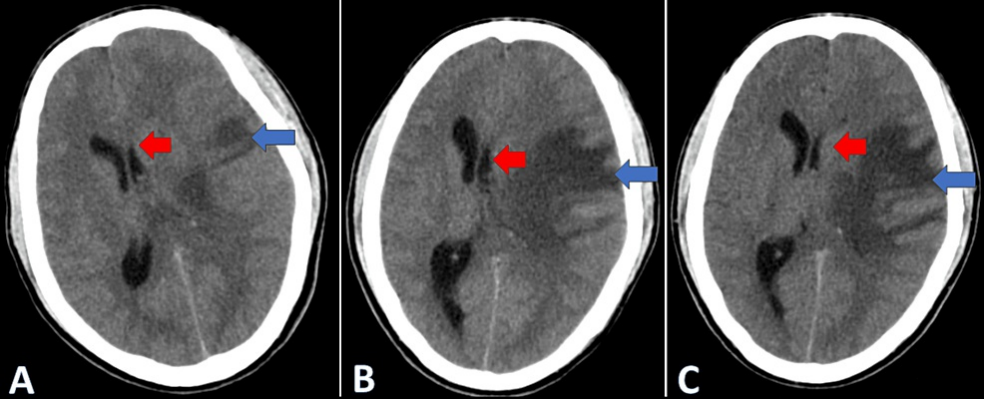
Serial CT brains (axial views) done on the third (A), seventh (B), and 10th (C) day shows the mass effect on the left lateral ventricle and a midline shift to the right side (red arrow) and effacement of surrounding sulci and gyri (blue arrow) due to cerebral edema CT: computed tomography
